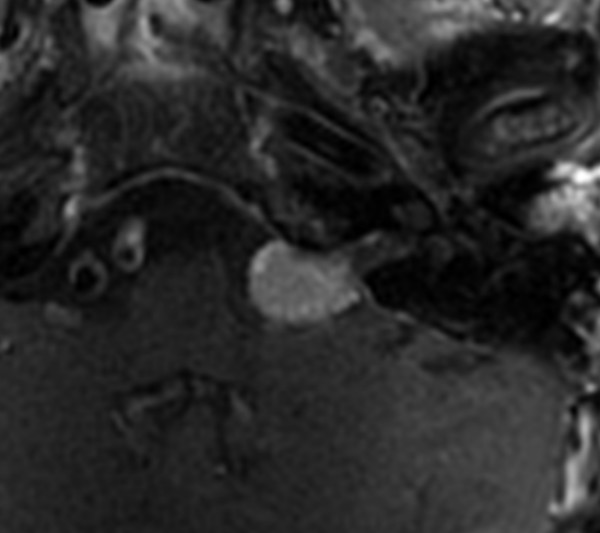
Post-treatment MRI. Most recent enhanced T1-axial MRI of the brain showing an enhancing lesion of the left cerebellopontine angle with a smaller maximum diameter than prior to gamma knife treatment.
